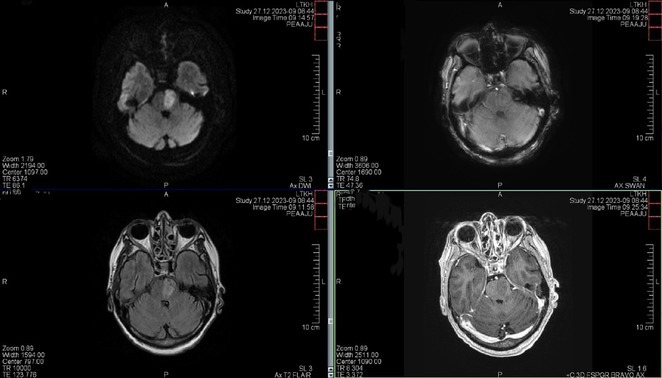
FIGURE 1 Second MRI scan showing ischemic lesion in the brainstem on the left.
